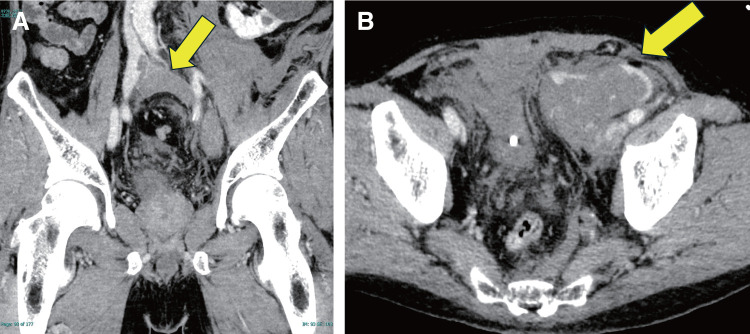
Computed tomography scans before surgery. (A) Venous thrombosis in the left iliac vein (arrow). (B) Hemorrhage in the retroperitoneal space (arrow).
